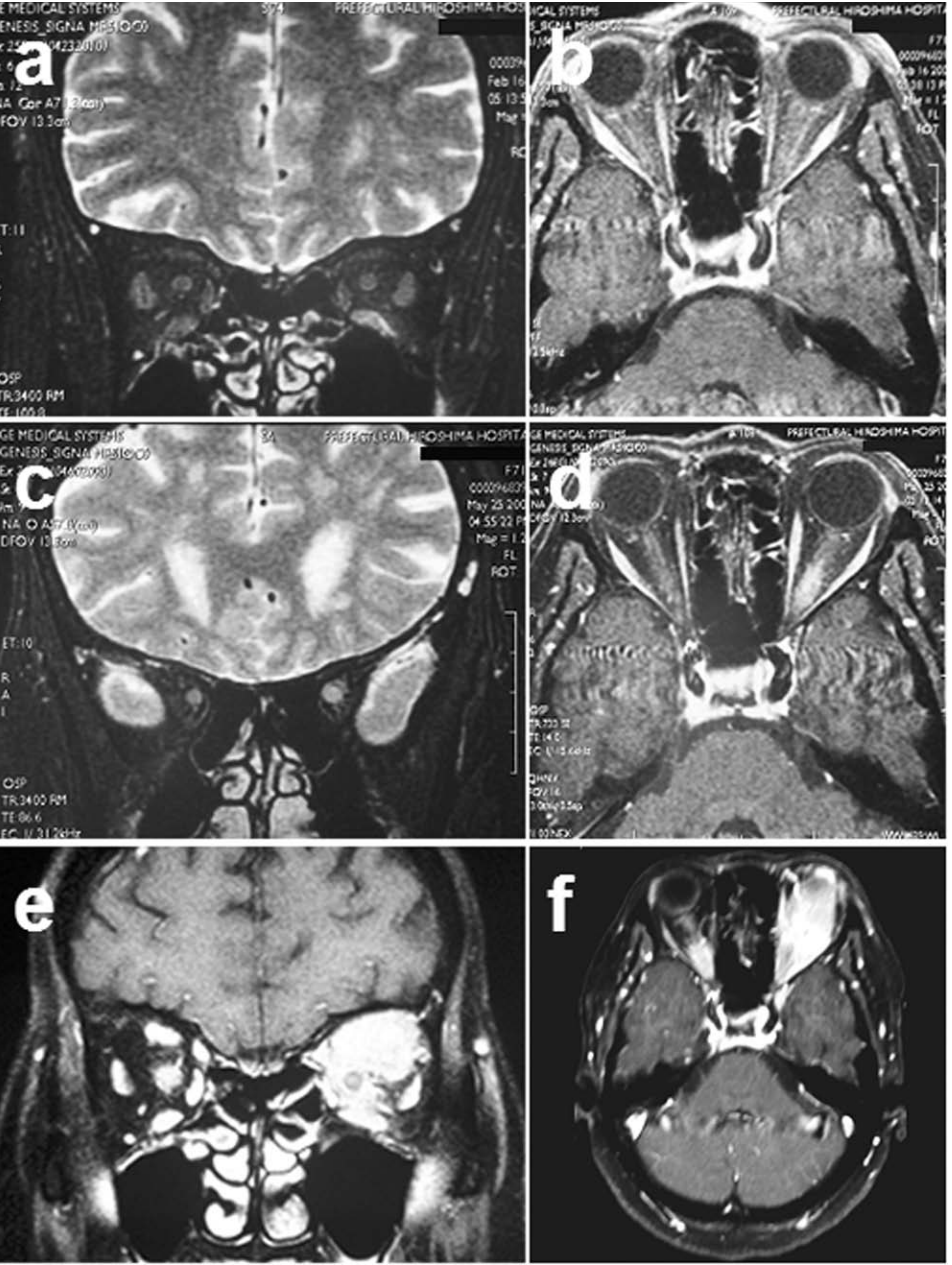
Magnetic resonance images of the head and orbits. (a) Initial coronal image (unenhanced T2-weighted) showing the left optic nerve to be thicker and more enhanced than the right optic nerve. (b) Transverse (enhanced T1-weighted) coronal image showing the left optic nerve to be thicker and more enhanced than the right optic nerve. (c) Coronal (enhanced T2-weighted) image obtained after loss of vision in both eyes, which shows that the right optic nerve has high signal intensity while the left optic nerve is swollen. (d) Transverse (enhanced T1-weighted) coronal image obtained after loss of vision in both eyes, which shows that the right optic nerve has high signal intensity while the left optic nerve is swollen. (e) Coronal (enhanced T1-weighted) image obtained six months after presentation displays bilateral orbital tumors. (f) Transverse (enhanced T1-weighted) coronal image obtained six months after presentation displays bilateral orbital tumors.
